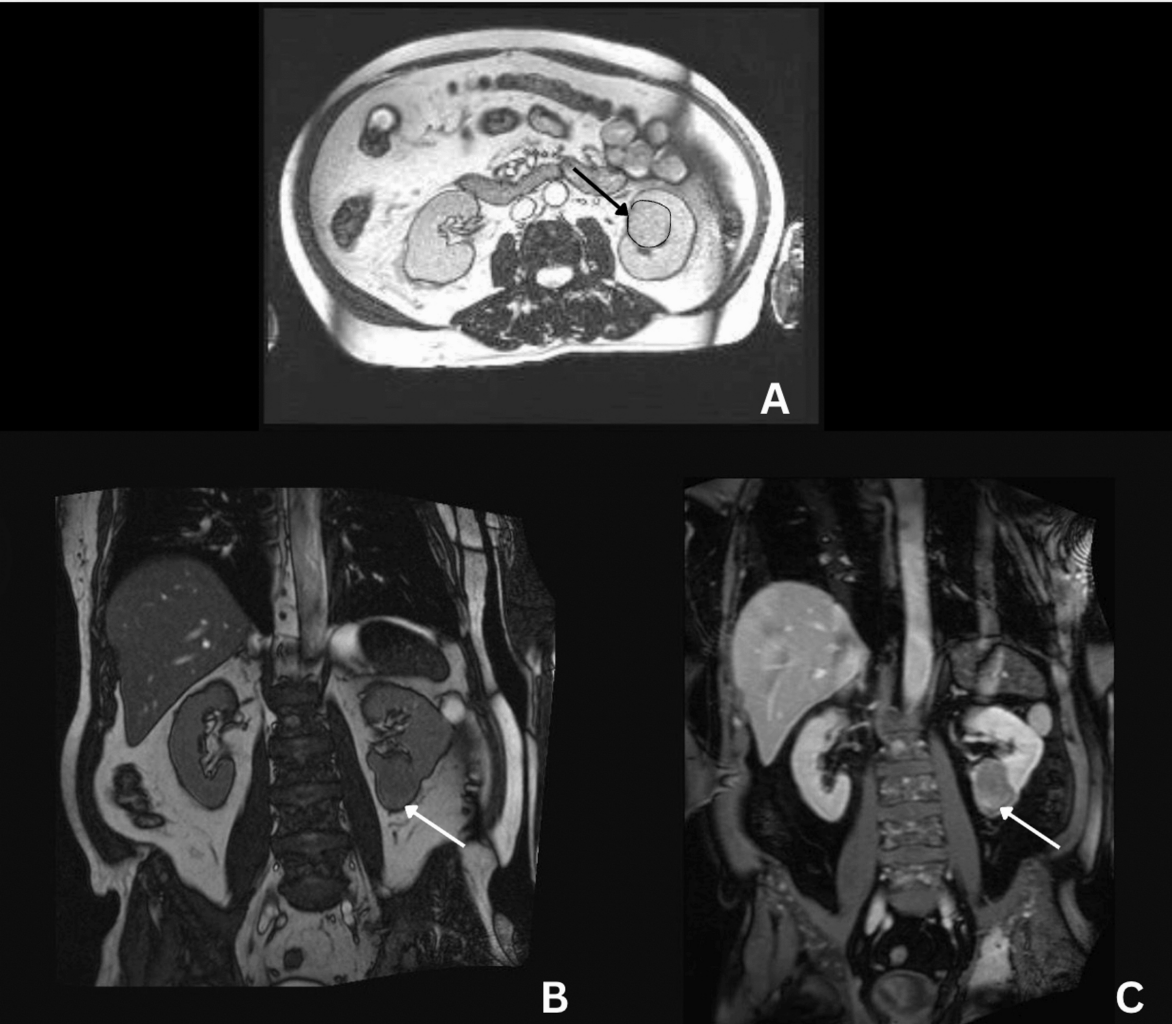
(A) Transverse T2-weighted abdominal and pelvic MRI. (B) Coronal T2-weighted abdominal and pelvic MRI. (C) Coronal T1-weighted post-contrast abdominal and pelvic MRI. All images reveal a suspicious left renal mass with multiple vertebral lytic lesions, suggestive of metastatic disease.
